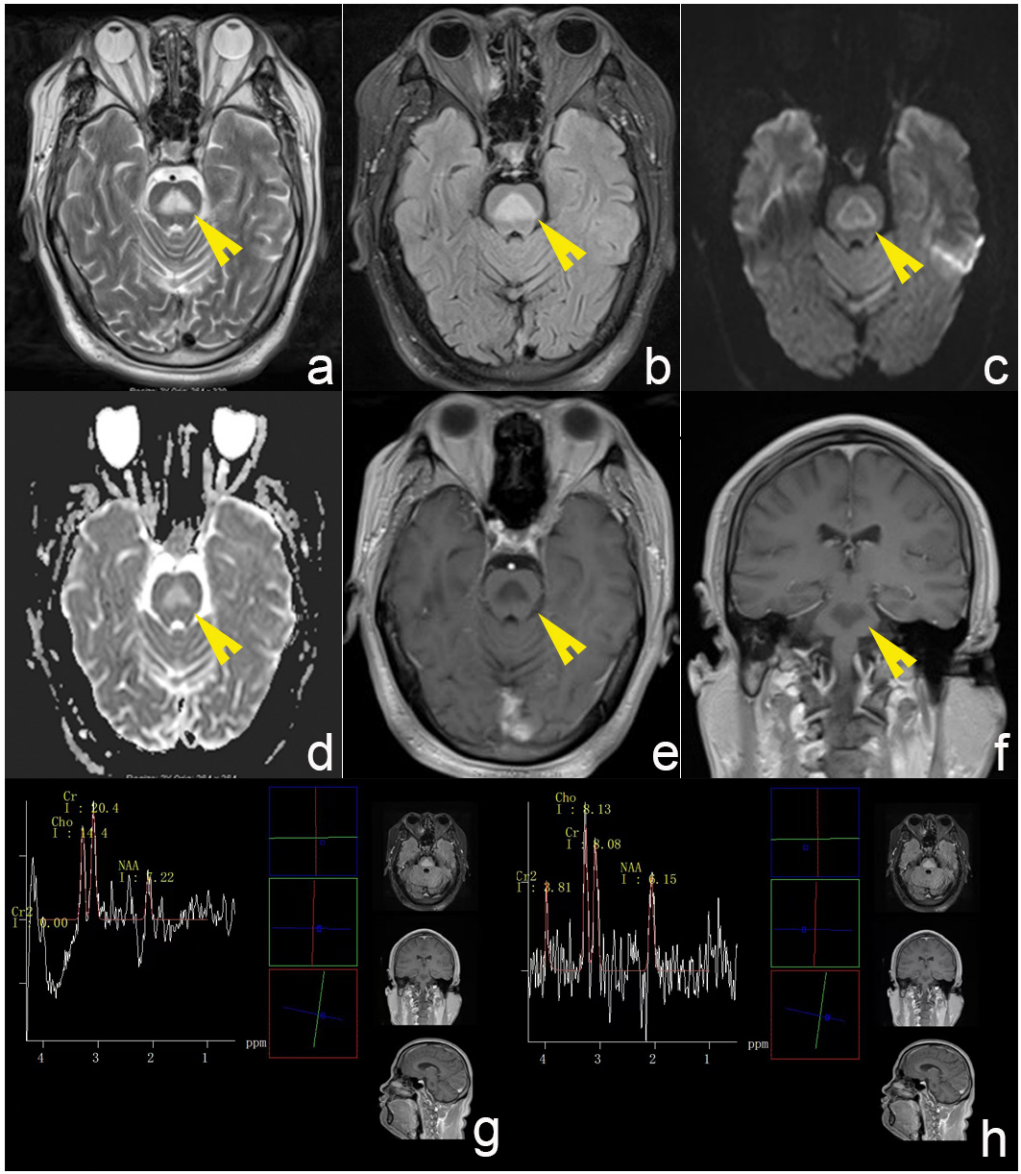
Brain MRI of the patient. (a,b) T2-weighted and FLAIR sequences revealed a triangular hyperintense signal within the central pons. (c) DWI revealed a markedly hyperintense rim surrounding the lesion. (d) ADC map showed corresponding hyperintensity in the lesion’s central region. (e,f) Post-contrast imaging revealed no areas of abnormal enhancement. The white arrows indicate lesion regions. (g,h) MRS indicated a significant decrease in N-acetylaspartate (NAA)/creatine (Cr) ratio and an increased choline (Cho)/Cr ratio. The yellow arrows denote diseased areas.
